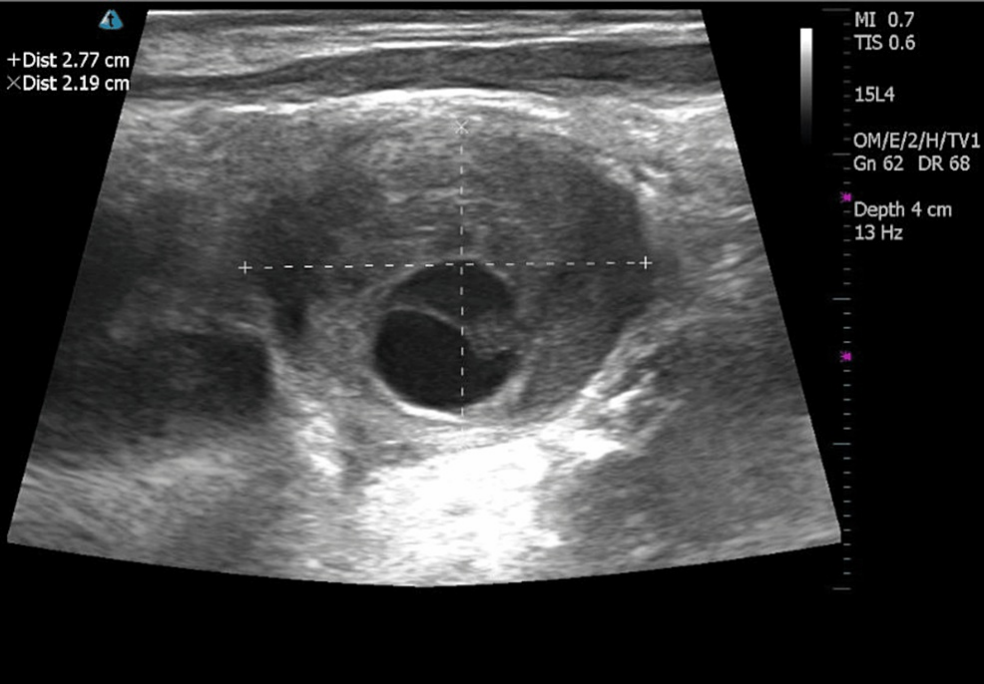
Two-dimensional ultrasound (2DUS) 13 months after sclerotherapy Two-dimensional ultrasound (2DUS) of patient’s left neck at his most recent follow-up visit, 13 months following sclerotherapy. The ultrasound image shows a significantly reduced left cervical lymphocele compared to pre-sclerotherapy measurements (Figure 2). There is a central cystic component with surrounding sclerosis secondary to ethanol therapy. This measures 2.77cm transversely (horizontal line) and 2.19 cm anteroposteriorly (vertical line).
